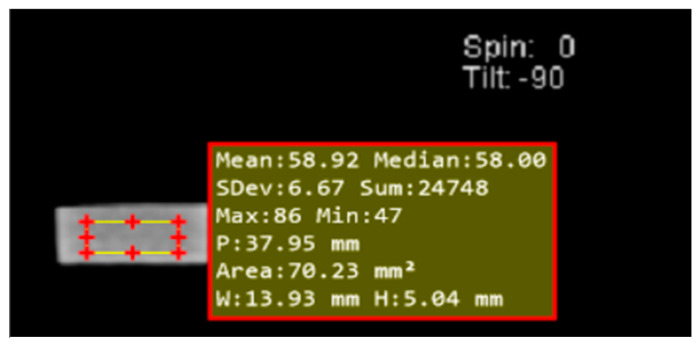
Example CT image of the cross-sectional area with the area of interest.
